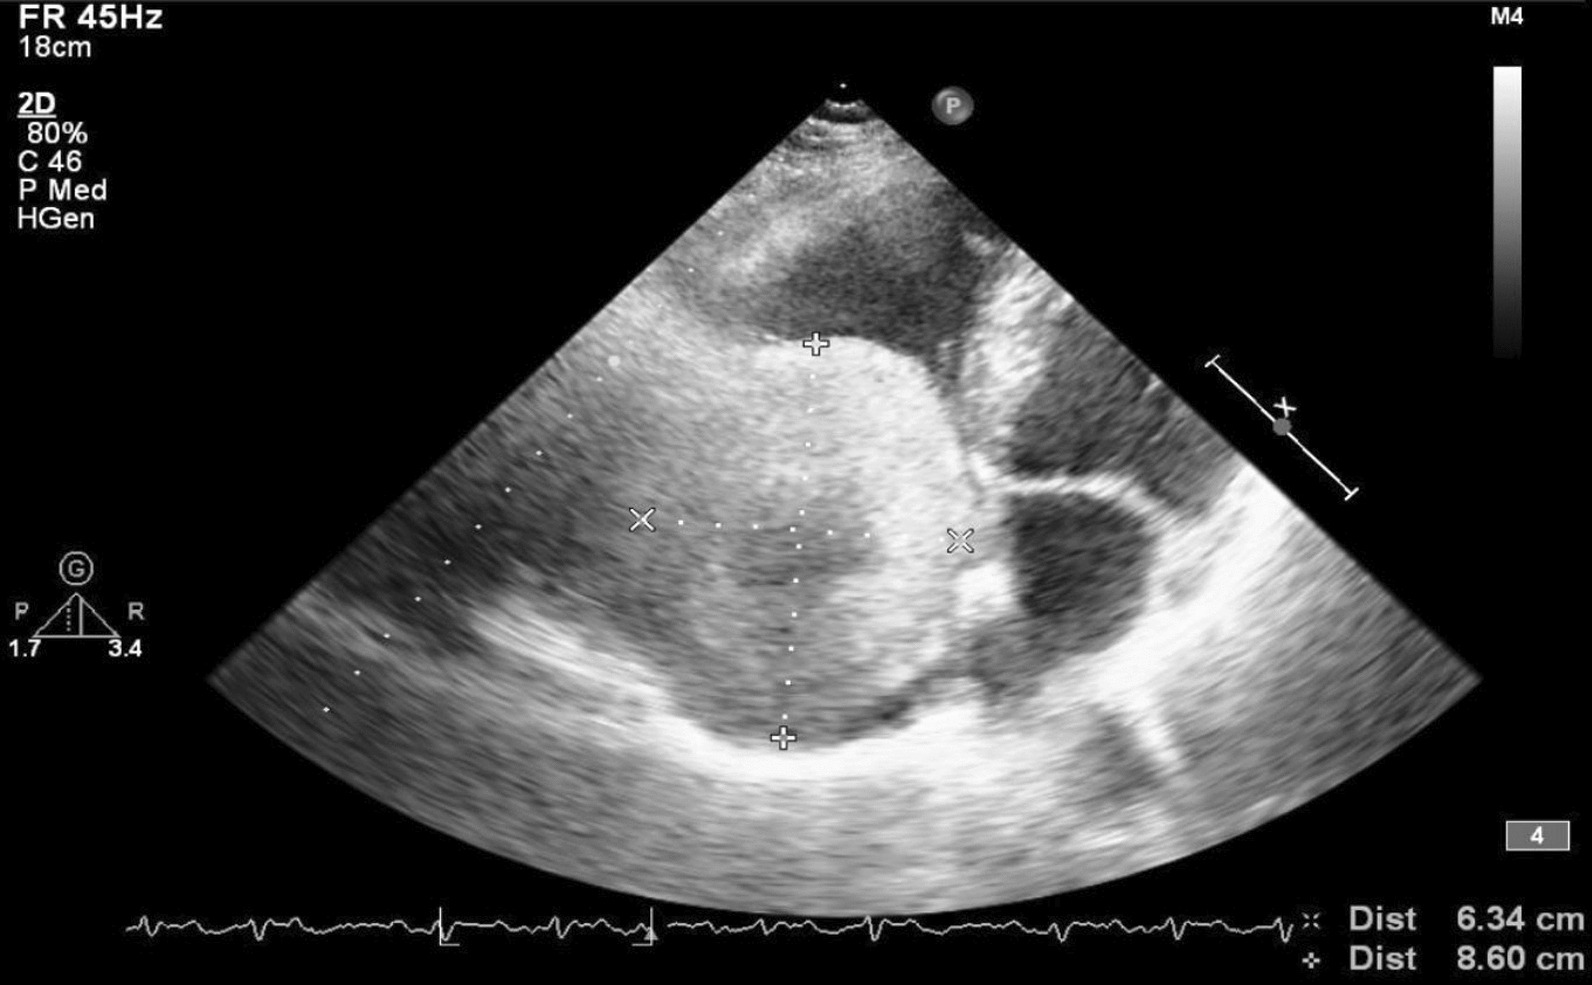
Modified apical four cavities view showing a huge intraatrial mass of 63 × 86 mm, with mechanical pressure on tricuspid valve and mild intrusion into the right ventricle
